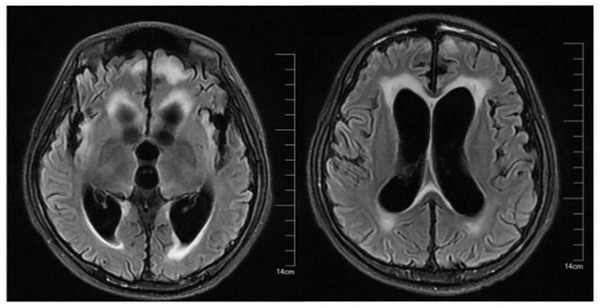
Postoperative cranial MRI
